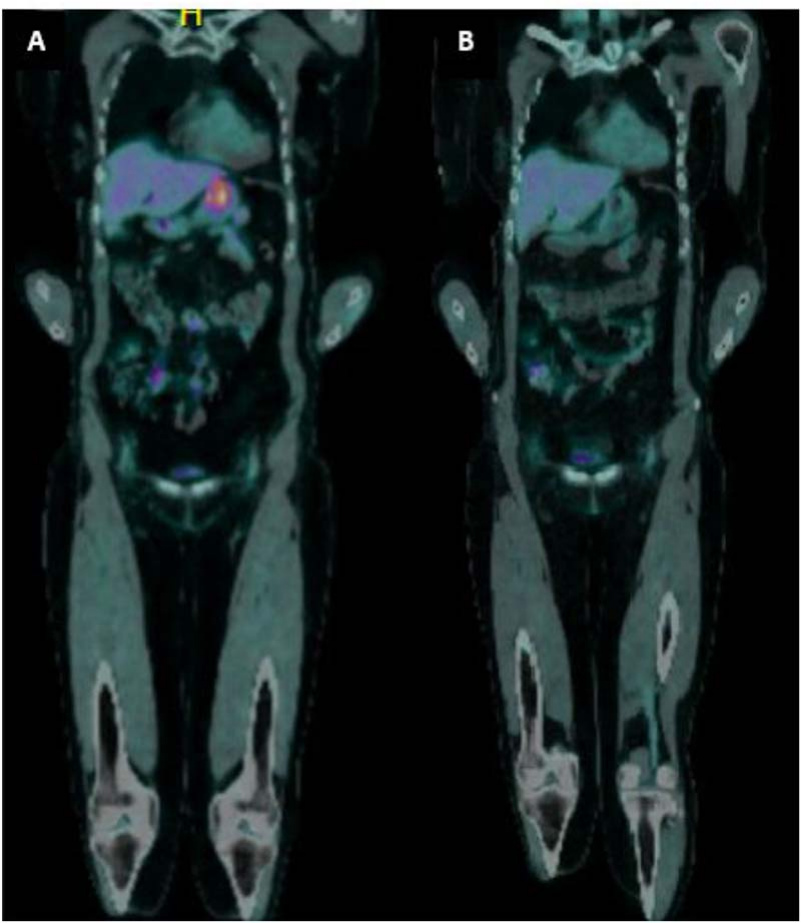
(a) PET imaging demonstrated intense FDG uptake along the lesser curvature of the stomach. (b) Interval PET imaging demonstrated reduced FDG uptake at the gastric lesser curvature and reduced gastric wall thickening.
